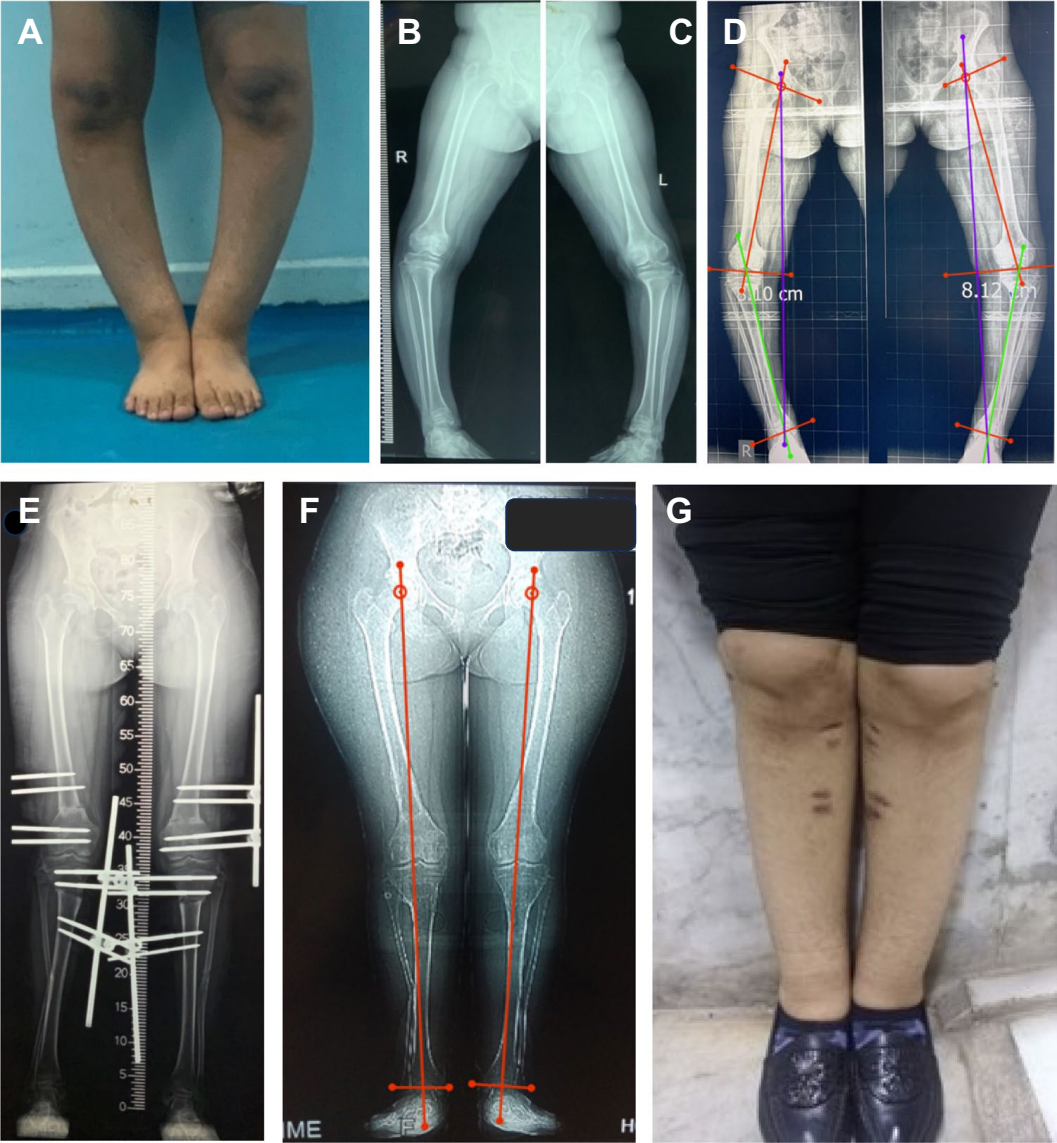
A A 14-year-old female patient with bilateral genu varum and internal tibial torsion. B, C Preoperative standing X-ray scanogram. D Preoperative measurement of joint orientation angles. E Postoperative scanogram after acute correction of the deformities using 2 monolateral frames. F, G Scanogram and clinical photo at the final follow-up after removal of the fixators
